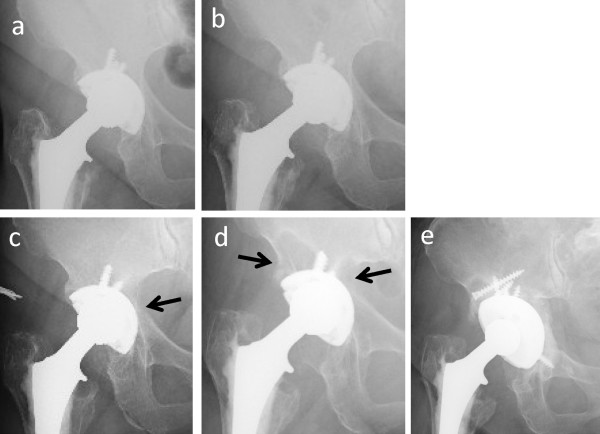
Serial radiographs of our patient. (a) A radiograph taken immediately after total hip arthroplasty in 1998 shows excellent incorporation of the cementless cup within the acetabulum. (b) A radiograph taken immediately after the accidental fall in 2008 shows no obvious fracture or osteolytic lesion. (c) A radiograph taken 1 year after the fall shows a small osteolytic lesion (arrow). (d) A radiograph on admission in 2010 shows an extended osteolytic lesion in the superolateral side of the ilium and the acetabulum (arrows) and no obvious migration of the cup or screws. (e) A post-operative radiograph taken in 2011 after resection of the hematoma lesion and cup revision surgery.
